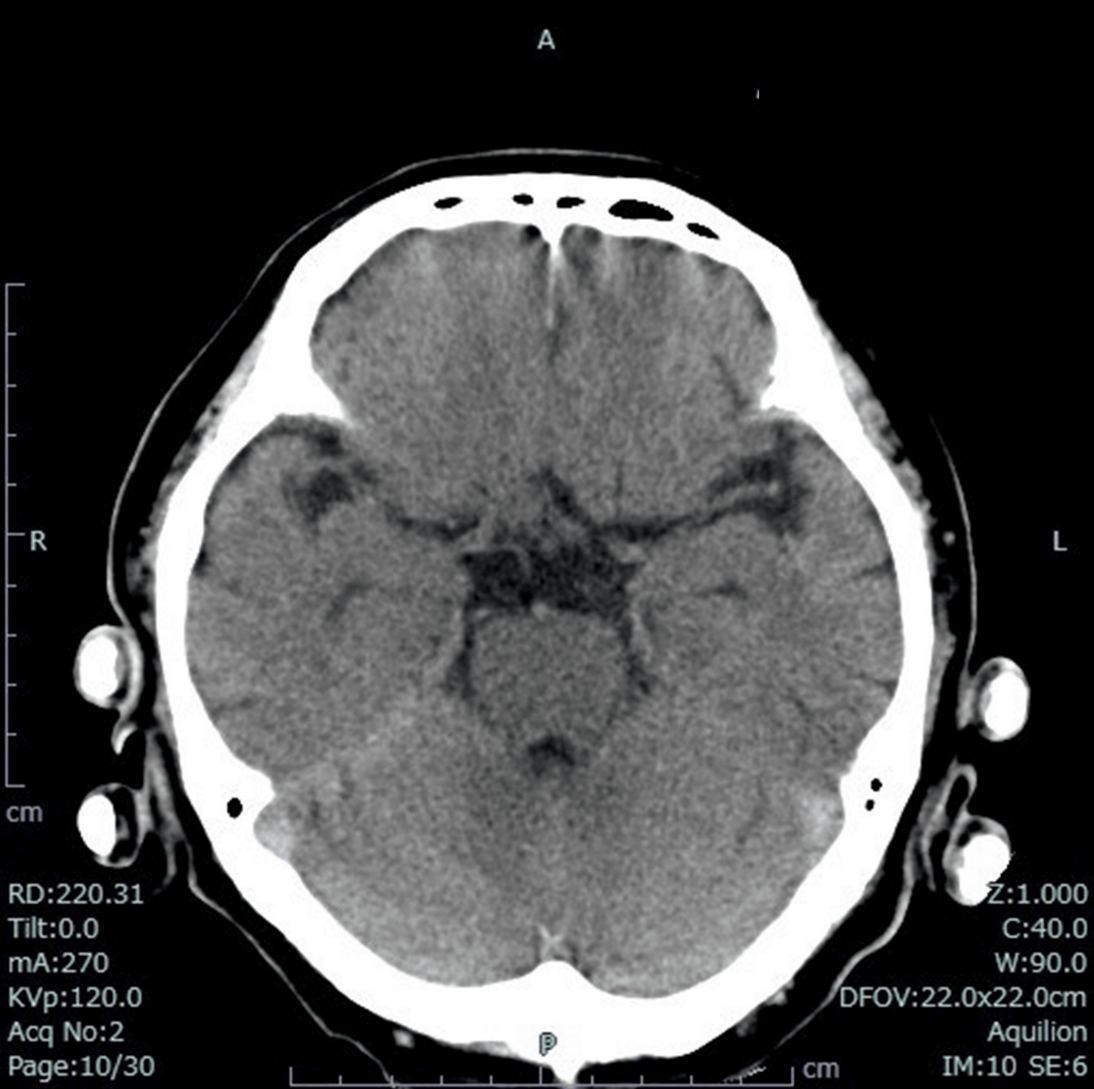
Head CT No abnormal findings.
